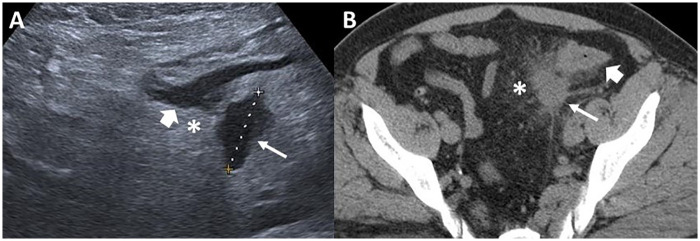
Acute left colonic diverticulitis stage 1b. A US image obtained with a convex transducer (A) shows a thickening of the wall of the colon (short arrow), hyperechogenic fat, and a liquid collection that results in abscess <4 cm. A contrast-enhanced CT in the axial plane (B) shows the same findings as US. A follow-up colonic endoscopy confirmed the presence of a single diverticulum at that location.
